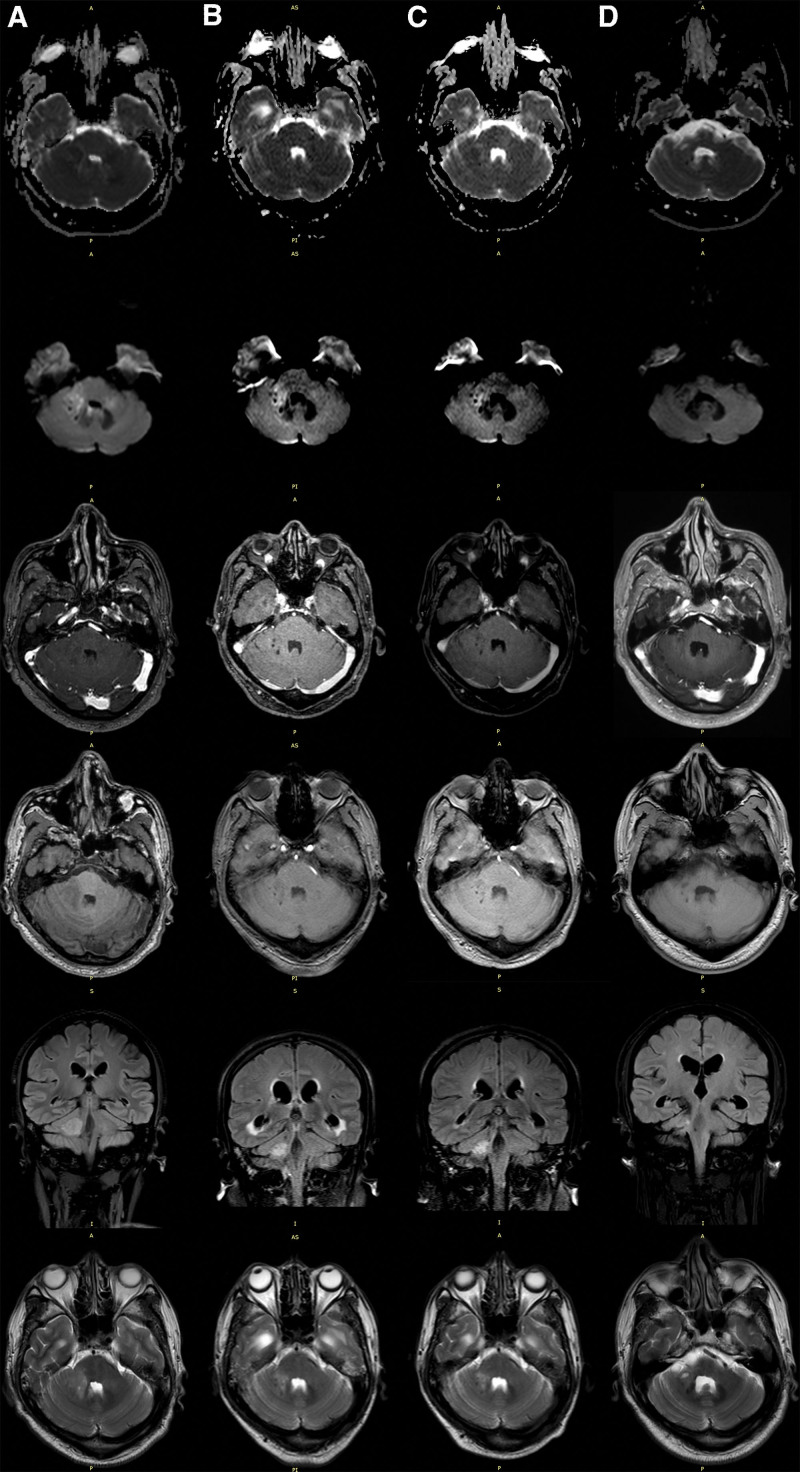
MRI of the head process. From top to bottom: DWI ADC, DWI b1000, T1W + C, T1W, T2 FLAIR, T2W. (A) After initial antibiotic treatment (September 14, 2022). The maximum length of the lesion on the right pons and brachium pontis is 2.9 cm, the maximum width of it is 2.5 cm, and the maximum circumference is 8.31 cm. The lesion signal: T1 slightly lower signal, with an average intensity of 222 (298); T2 high signal, with an average intensity of 463 (285); FLAIR high signal, with an average intensity of 711 (619); DWI slightly higher signal, with an average intensity of 352 (239); ADC slightly higher signal, with an average strength of 705 (654). The number of visible layers of the lesion is 4 (slice thickness = 5 mm). The lesion is not significantly enhanced but we can see local thickening of the left meninges with enhancement. (B) After 1 month Antibiotic Modification Treatment (October 11, 2022). The maximum length of the lesion is 2.5 cm, the maximum width of it is 2 cm, and the maximum circumference is 6.80 cm. The lesion signal: T1 slightly lower signal, with an average intensity of 168 (186); T2 high signal, with an average intensity of 628 (409); FLAIR slightly higher signal, with an average intensity of 374 (280); DWI slightly higher signal, with an average intensity of 351 (221); ADC high signal, with an average strength of 1116 (720). The number of visible layers of the lesion is 2 (slice thickness = 5 mm). The lesion is not significantly enhanced. The figure shows a significant widening of the supratentorial ventricle of the cerebellum. (C) On second admission (November 29, 2022). The maximum length of the lesion is 2 cm, the maximum width of it is 1.2 cm, and the maximum circumference is 4.94 cm. The lesion signal: T1 slightly lower signal, with an average intensity of 180 (199); T2 high signal, with an average intensity of 645 (471); FLAIR high signal, with an average intensity of 367 (263); DWI slightly higher signal, with an average intensity of 149 (138); ADC high signal, with an average strength of 807 (611). The number of visible layers of the lesion is 2 (slice thickness = 5 mm). The lesion is not significantly enhanced. The figure shows a significant widening of the supratentorial ventricle of the cerebellum. (D) On third admission (July 5, 2023). The maximum length of the lesion is 1.75 cm, the maximum width of it is 0.54 cm, and the maximum circumference is 1.91 cm. The lesion signal: T1 slightly lower signal, with an average intensity of 273 (356); T2 high signal, with an average intensity of 673 (359); FLAIR slightly higher signal, with an average intensity of 367 (284); DWI liquefaction necrosis low signal, with an average intensity of 57 (118), ADC high signal, with an average intensity of 1856 (906). The number of visible layers of the lesion is 1 (slice thickness = 5 mm). The lesion is not significantly enhanced. The figure shows a significant widening of the supratentorial ventricle of the cerebellum. Due to the lack of original MRI images in the external hospital 5 days before admission, we provide the description of the MRI result. The results indicate abnormal signals in the right pons and brachium pontis; the parentheses after the “average intensity” represent the average intensity of the surrounding tissue of the lesion, serving as a comparison.
